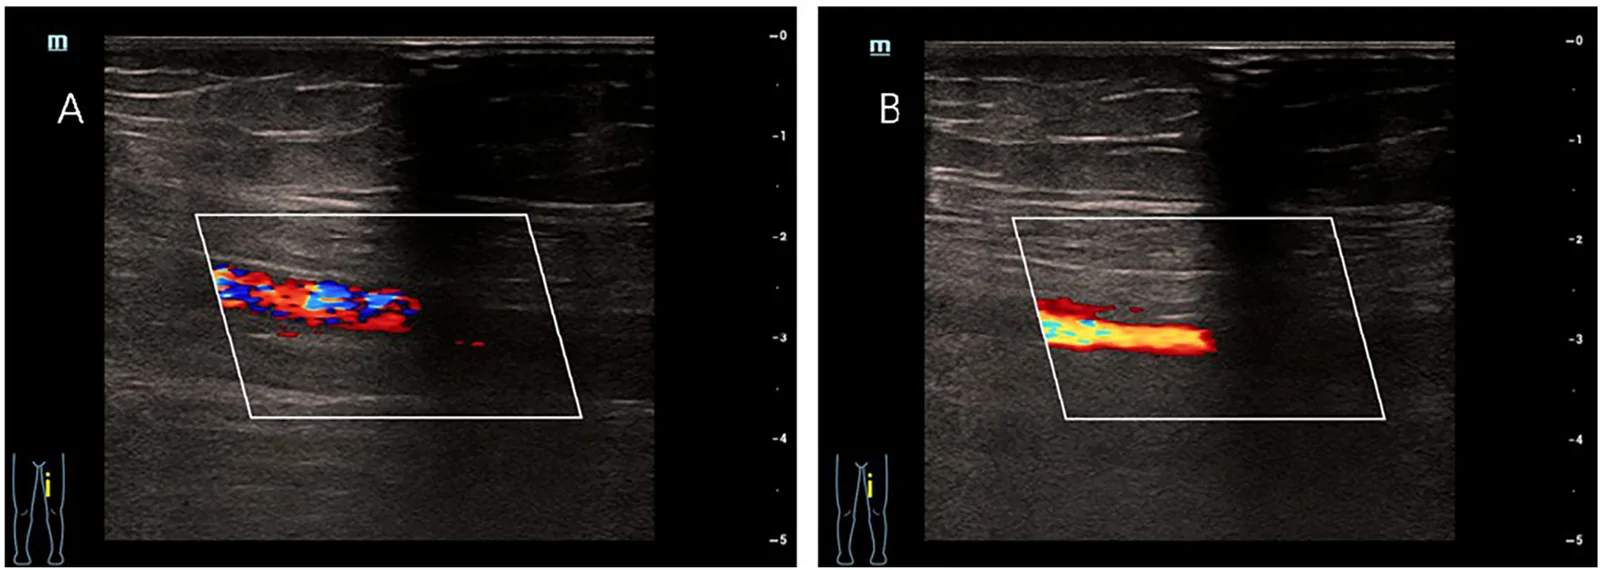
Repeat sonogram of hematoma.
